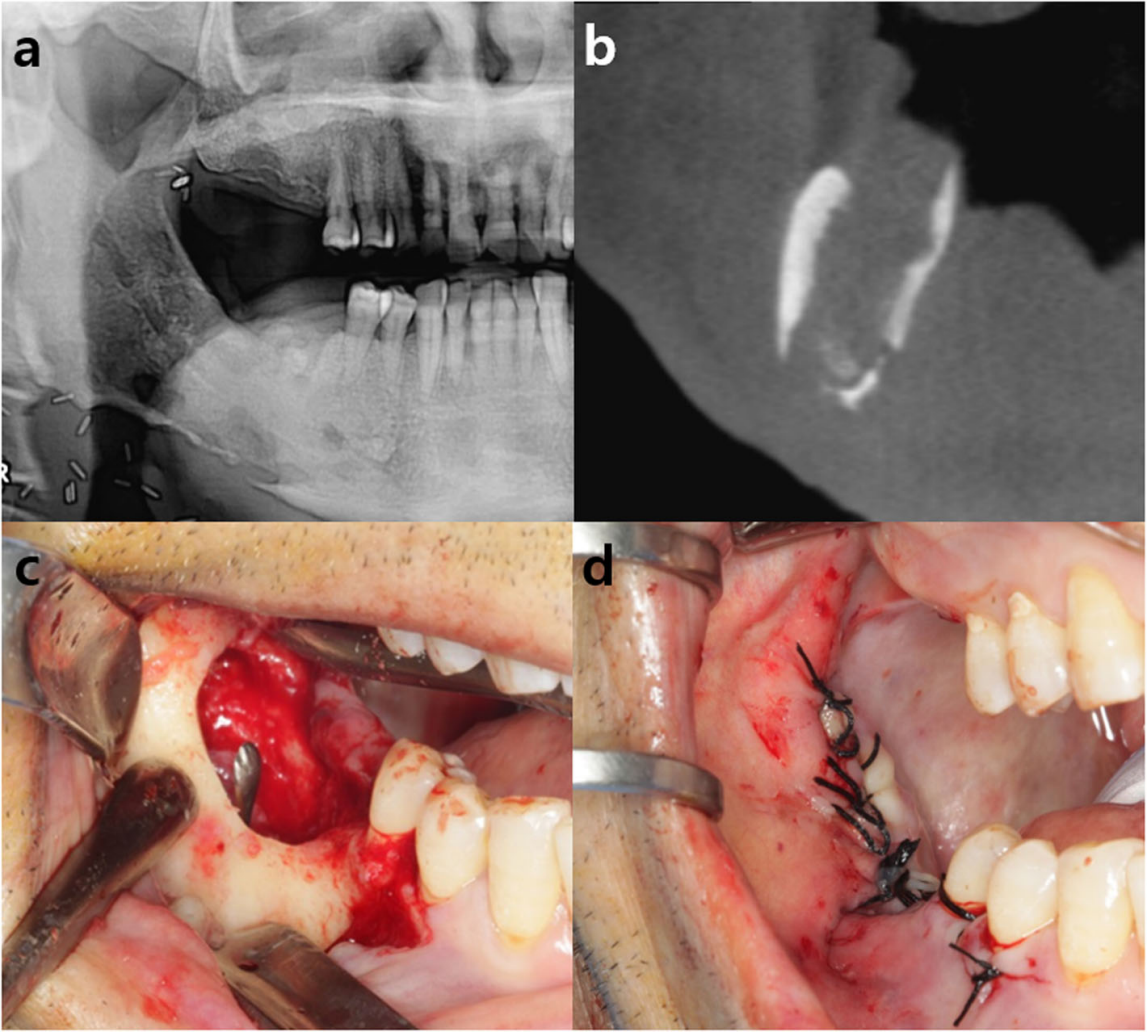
a, b Preoperative panoramic view and CT view showing partial osteonecrosis on the right mandibular body and angle. c Intra-oral photograph showing communicated fistulas on the intra- and extra-oral cavity after sequestrectomy via intra-oral approach. d The communicated fistulas on the intra- and extra-oral cavity were sutured for primary closure
